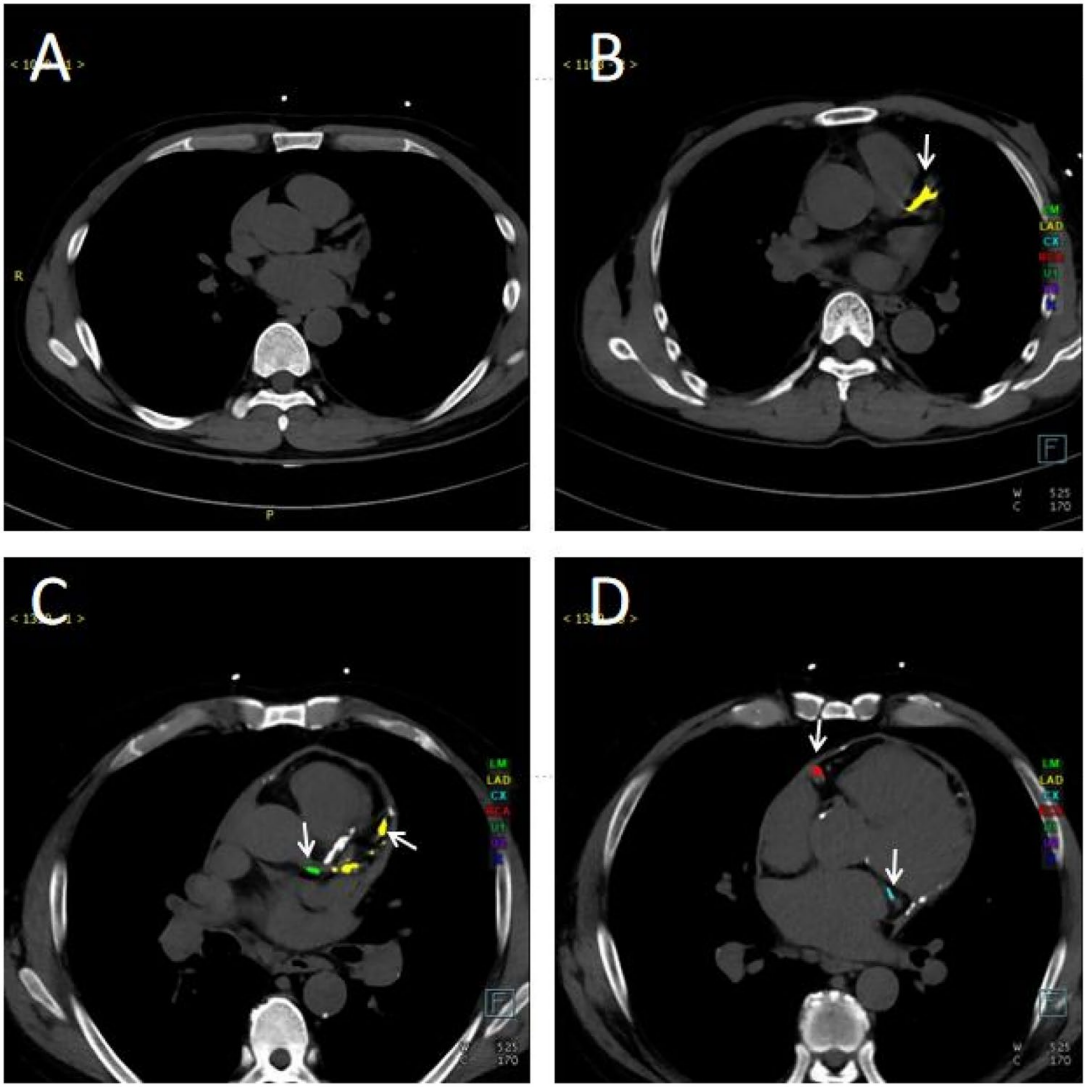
Cross-sectional CT images of ESKD patients with varying degrees of CACS. (A) An ESKD patient without coronary calcification. (B) An ESKD patient with calcification only in the LAD. (C–D) An ESKD patient with calcification in all four coronary branches. (C) Calcification in the left main trunk and left anterior descending branch. (D) Calcification in the circumflex branch and right coronary artery. Different colors represent each coronary branch with calcification (green: left main trunk; yellow: left anterior descending branch; blue: circumflex branch; red: right coronary artery), and the arrows indicated the calcified branches. Abbreviations: CT: computed tomography; ESKD: end-stage kidney disease; LAD: left anterior descending branch.
